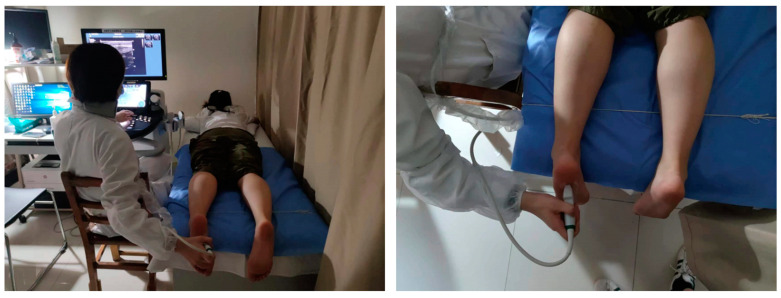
Acquisition of ultrasonic images.
